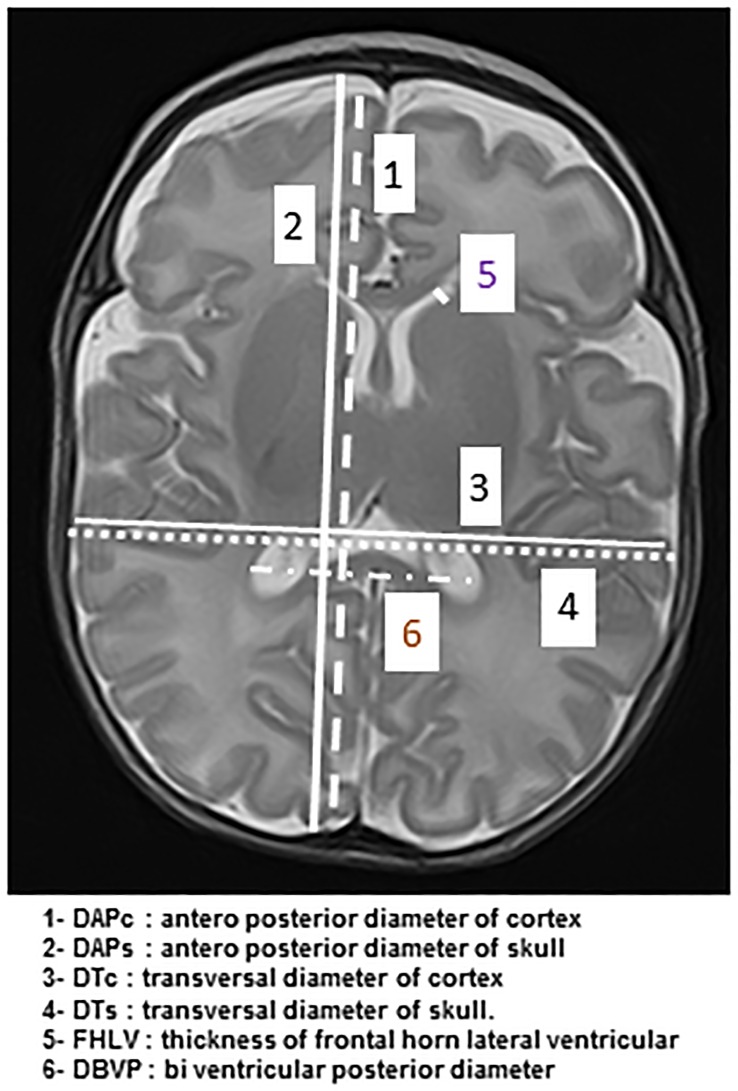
MRI linear indices on the axial image in T2-weighted including deep nuclei gray matter and Monro’s foramens. DAPc: anterior-posterior diameter of cortex /DAPs: anterior-posterior diameter of skull/ DTc: transversal diameter of cortex / DTs: transversal diameter of skull/ FHLV: thickness of frontal horn of lateral ventricle/ DBVP: biventricular posterior diameter.
